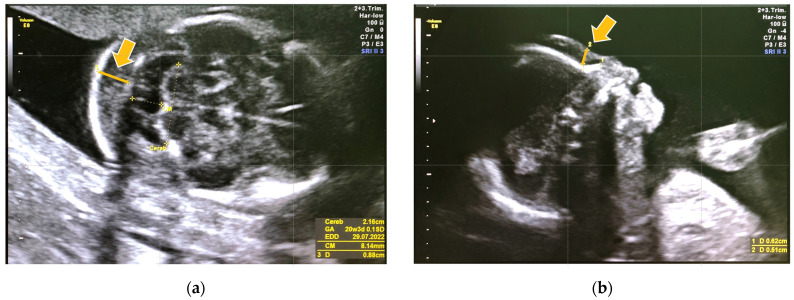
Prenatal ultrasonography of case 2 in the second trimester. (a) Nuchal fold thickness is the area of tissue at the back of the fetus (indicated by an arrow). (b) Prenasal thickness is the shortest distance between the anterior edge of the lowest part of the frontal bone and the facial skin anteriorly (indicated by an arrow). Both of them were increased.
